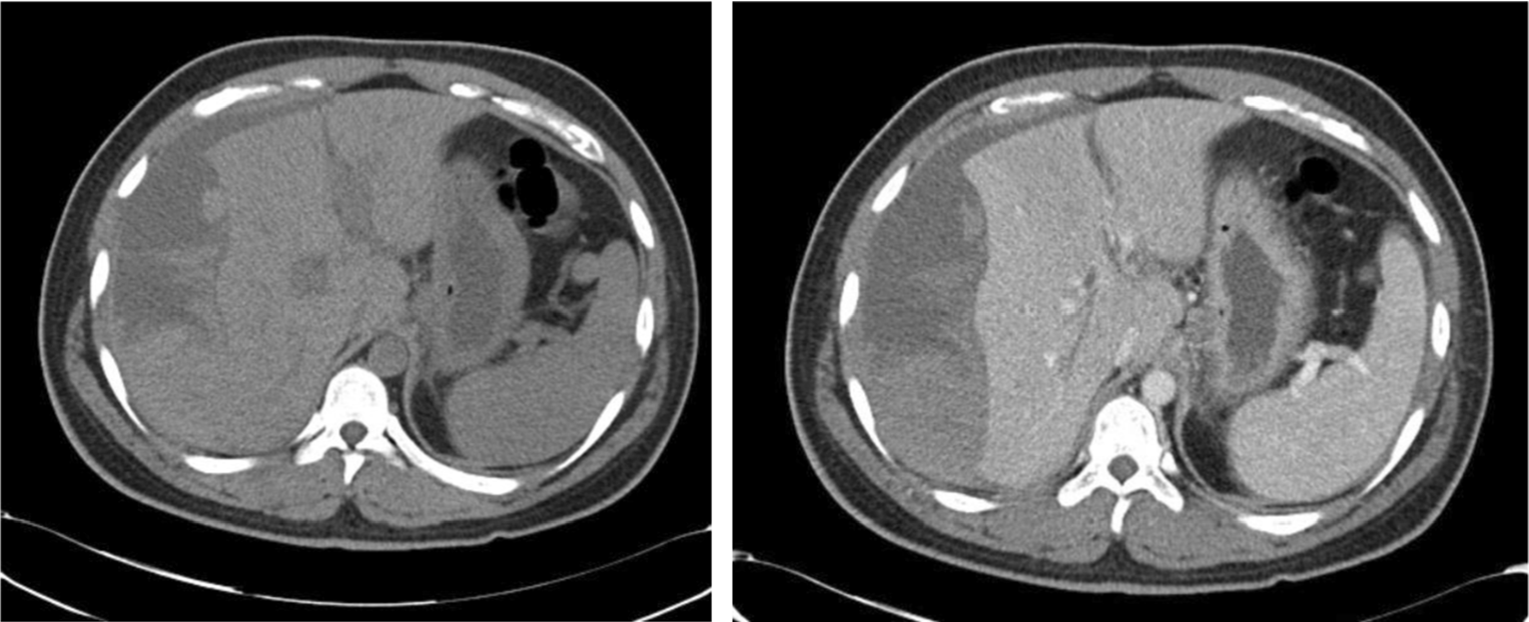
Non-contrast CT (*left*) showing a heterogenous subcapsular haematoma indenting the liver post liver biopsy. There is no enhancement or active bleeding on portal venous phase (*right*). Note the heterogeneity of the lesion with serum being anti-dependent, and more solid components of the haematoma being dependently positioned
